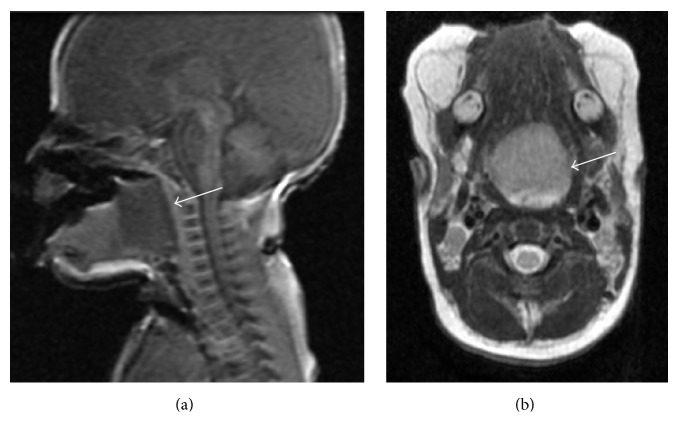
MRI findings of the mass on the tongue base. MRI shows a well-circumscribed mass measuring 25 × 23 × 27 mm in size in the midline region of the tongue base with a low signal intensity on T1-weighted images (a) and a high signal intensity on T2-weighted images (b).
